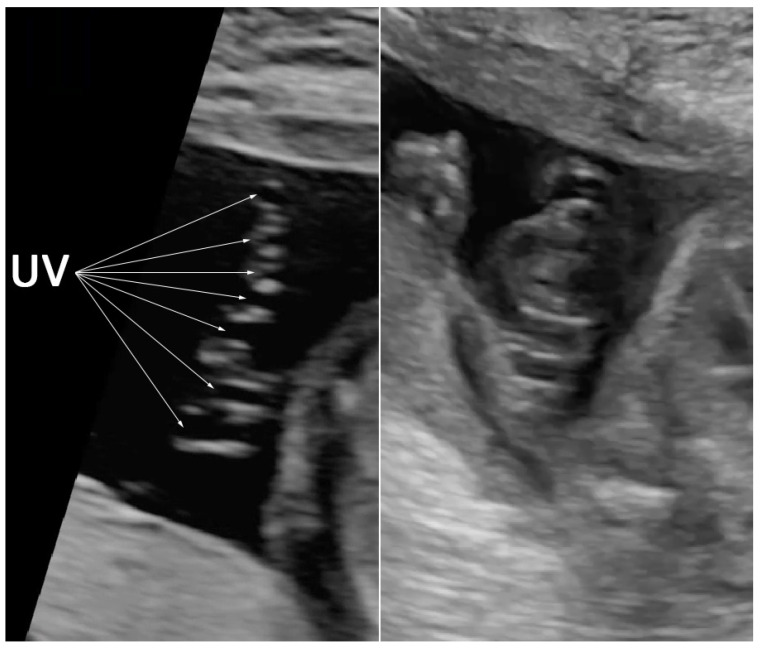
Prenatal ultrasound shows hypercoiling of the umbilical cord (7 loops in a 6 cm length) (UV: umbilical vein).
